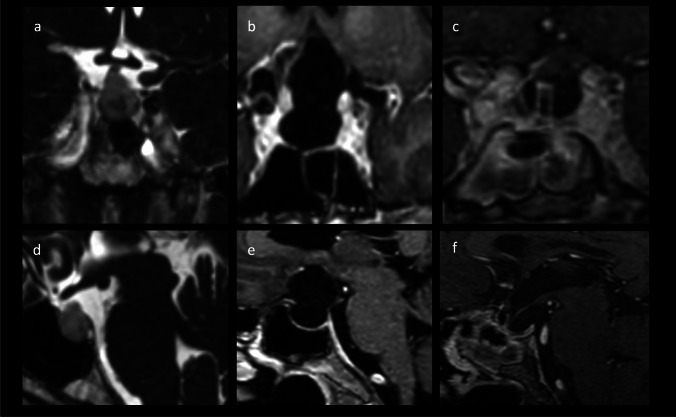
Exemplary case of a patient with cyst progression after initial WWS. a, d RCC without optic nerve compression at initial diagnosis on MRI CISS sequence. b, e Contrast-enhanced T1 sequences with evidence of cyst progression 144 months after initial diagnosis. c, f Postoperative contrast-enhanced T1 sequences without signs of residual cyst
